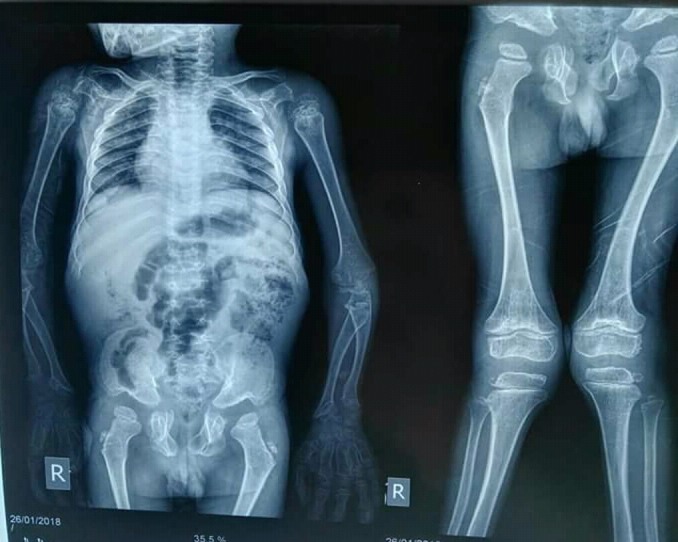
X-ray showed genu valgum in lower extremities with bowing of lower legs. No scoliosis was observed.
